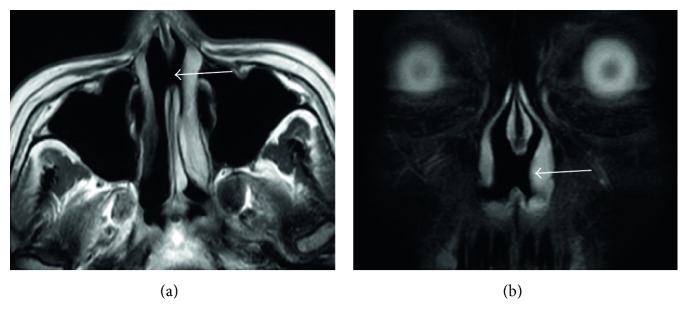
Magnetic resonance imaging (MRI) findings. MRI shows nasal septal perforation in horizontal (a) and coronal (b) sections.
